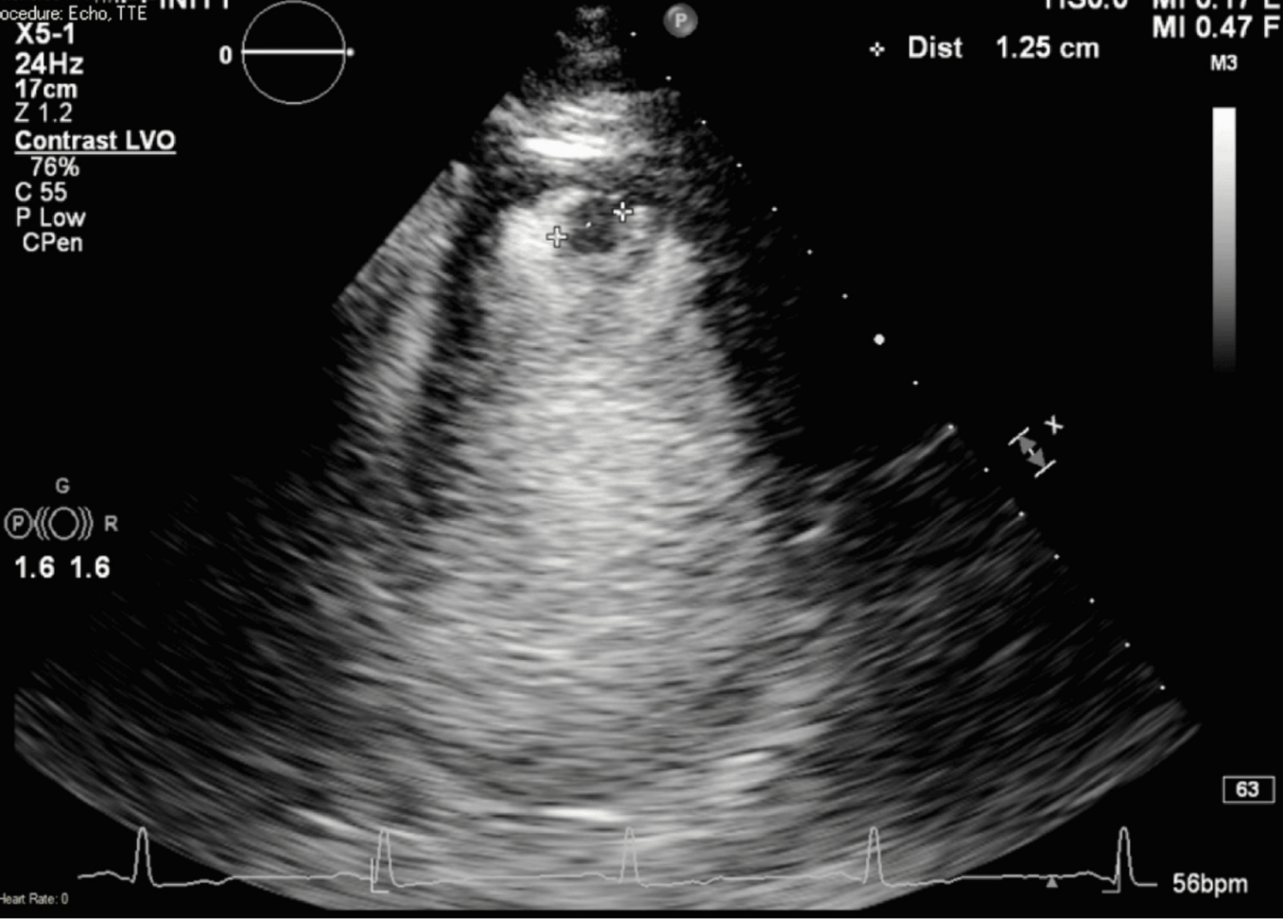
Transthoracic echocardiography showing evidence of mural apical filling defect consistent with left ventricular apical thrombus.
